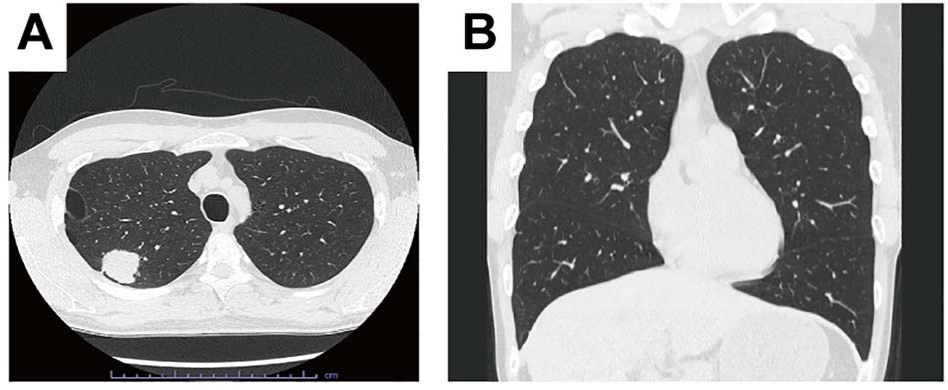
Computed tomography showed a 33 mm mass in the right upper lobe. A. No interlobar fissure between the right upper lobe and middle lobe
